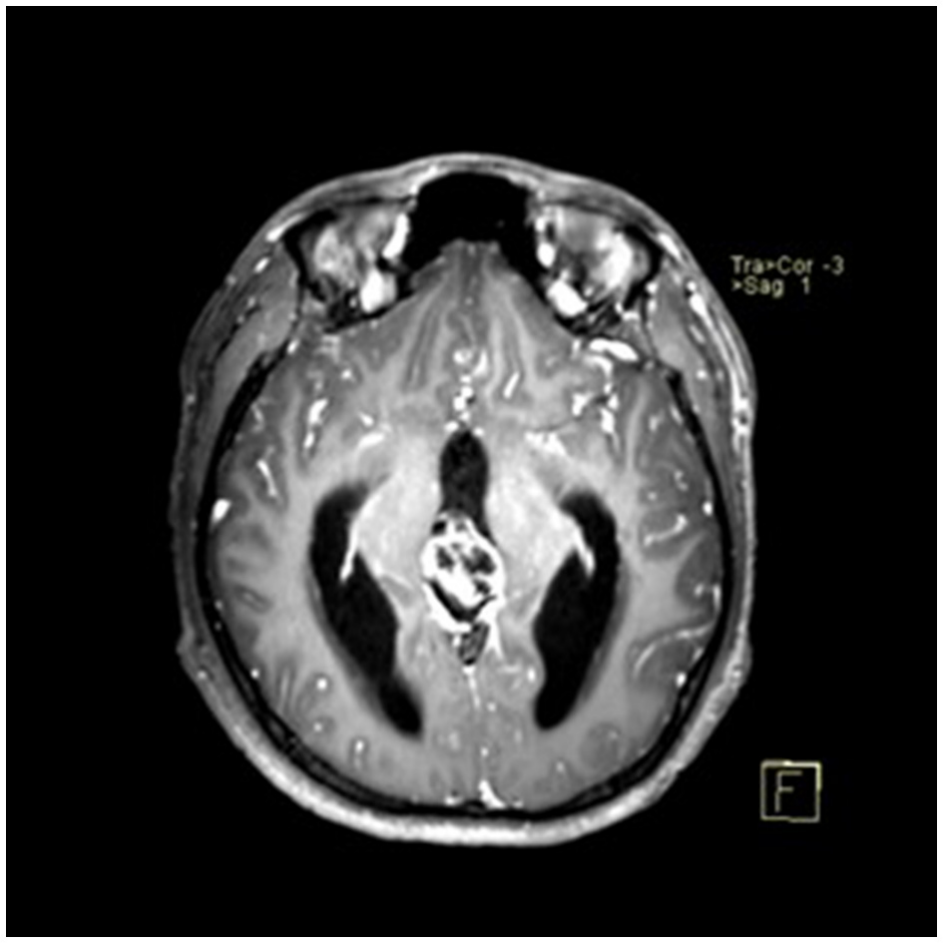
Preoperative contrast-enhanced MRI (axial view) showing a cystic-solid mass in the pineal region with supratentorial hydrocephalus.
